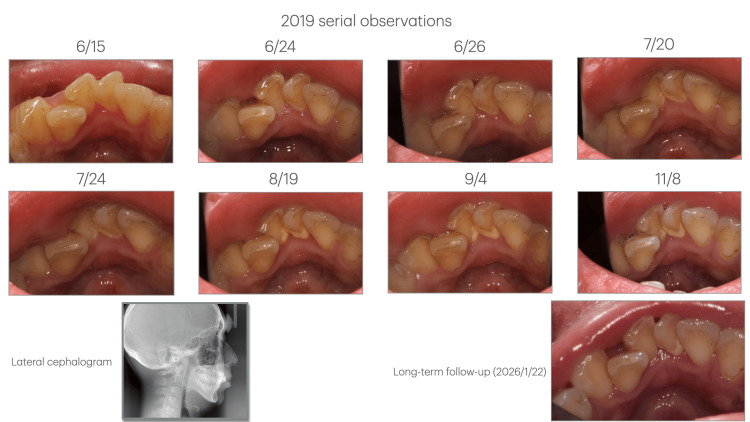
Serial intraoral observations in 2019 and long-term follow-up findings in Case 2 Upper two rows: serial intraoral photographs obtained in 2019, showing gradual re-accumulation of calculus on the lingual surfaces of the mandibular anterior teeth over time after scaling. Lower left: radiographic image obtained prior to the 2019 observation period. Lower right: long-term follow-up intraoral photograph, showing no clear renewed progressive enlargement compared with the earlier plateau-like stage.
